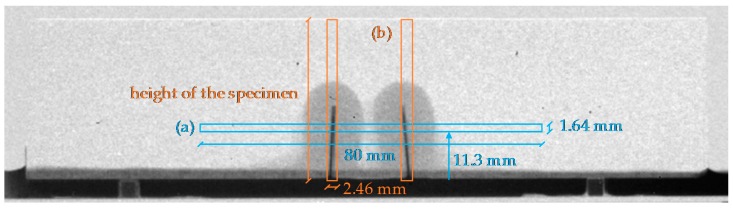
Location and dimensions of the rectangular areas for water profiling in a sample neutron radiography image: (a) perpendicular to the cracks; and (b) coinciding with the cracks.
